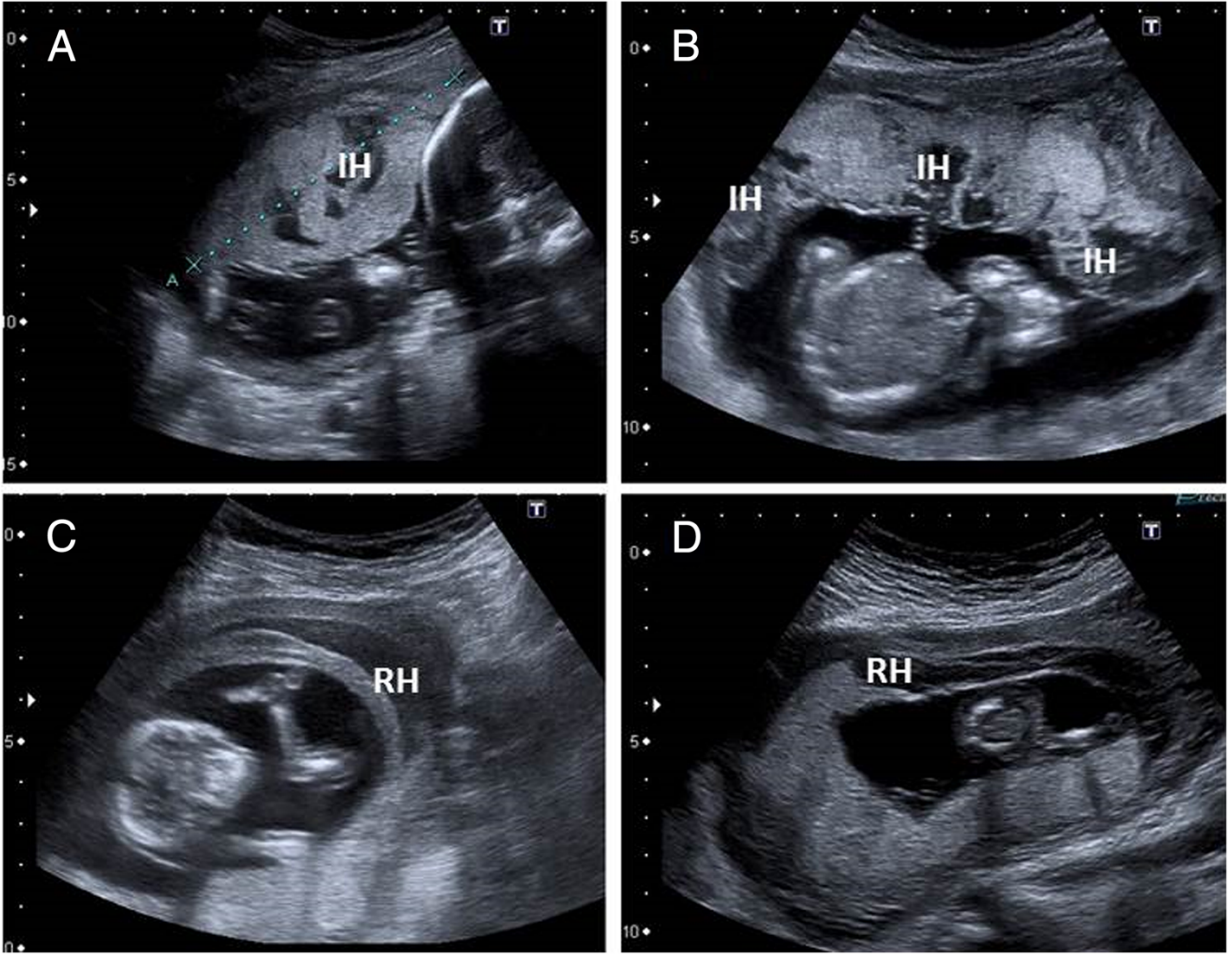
Sonographic appearance of intraplacental hematomas (“IH”, a and b) and retroplacental hematomas (“RH”, c and d)
